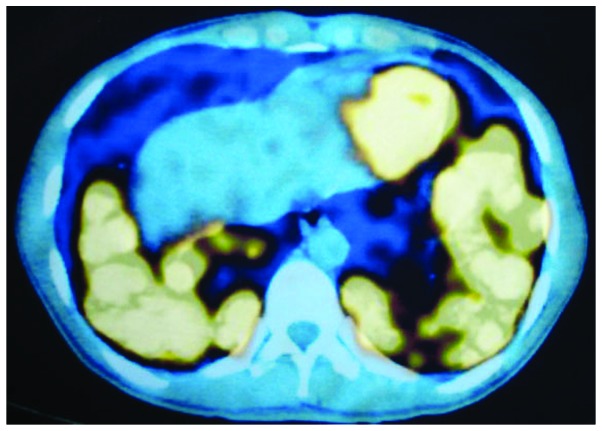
Computed tomography-positron emission tomography (CT-PET) image demonstrating multiple hypermetabolic pulmonary nodules.
